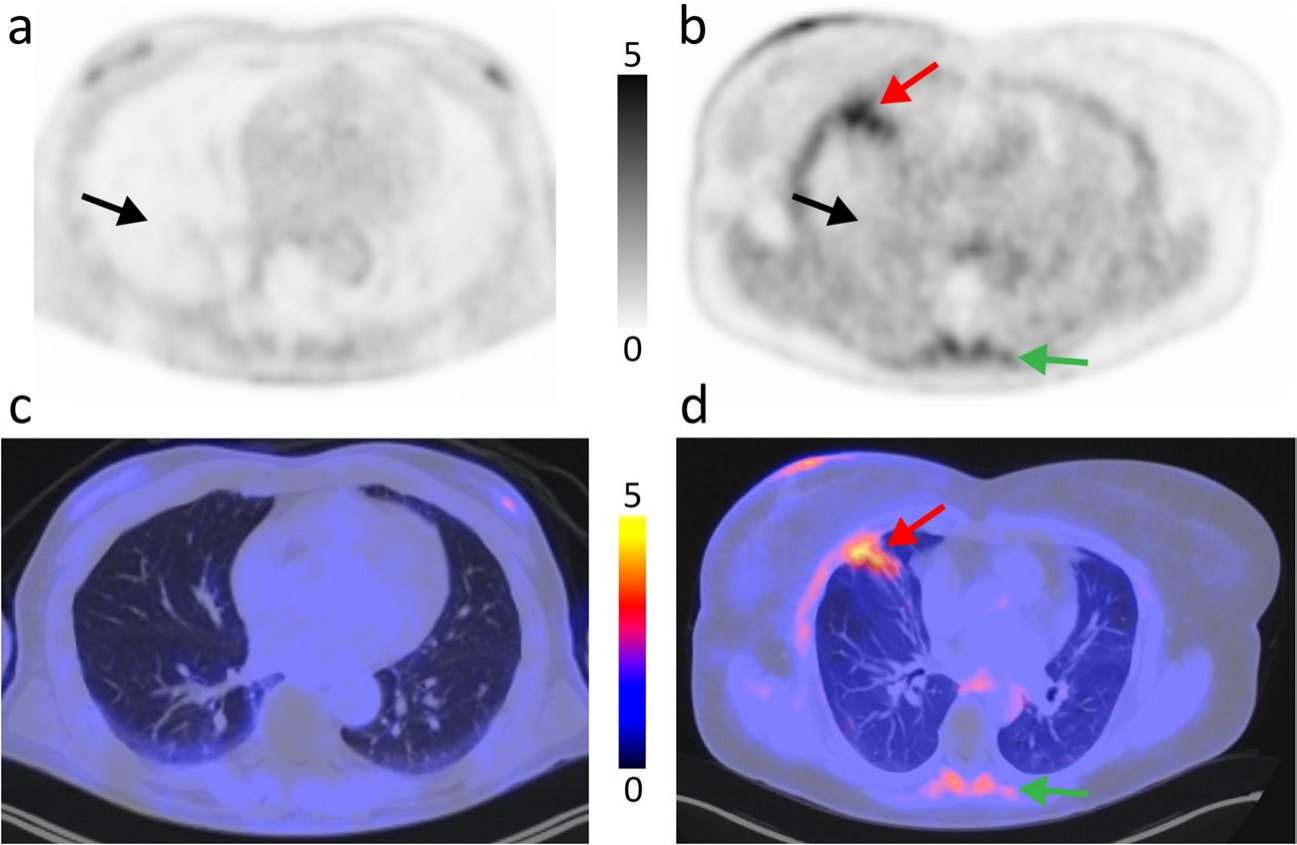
[^68^Ga]FAPI PET/CT scan of a PACS patient and control. EARL 2 reconstructed. A Transversal pulmonary PET image of a control patient. B Transversal pulmonary PET image of a PASC patient. C Transversal pulmonary fusion PET/CT image of a control patient. D. Transversal pulmonary fusion PET/CT image of a PASC patient. Black arrow: lung tissue, representative for diffuse [^68^Ga]FAPI uptake in the lungs. Red arrow: focal [^68^Ga]FAPI uptake in a fibrotic lesion. Green arrow: [^68^Ga]FAPI uptake in the paravertebral muscles. Settings: SUV 0–5, low-dose CT W 1200, C −600. Fusion 50/50
